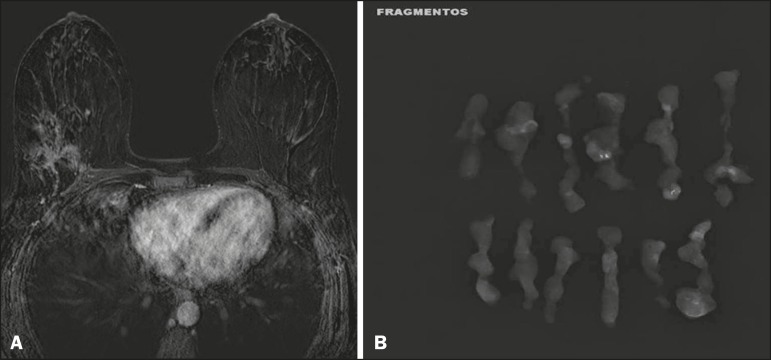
High-resolution MRI of the breasts. A: Axial sequence, with digital
